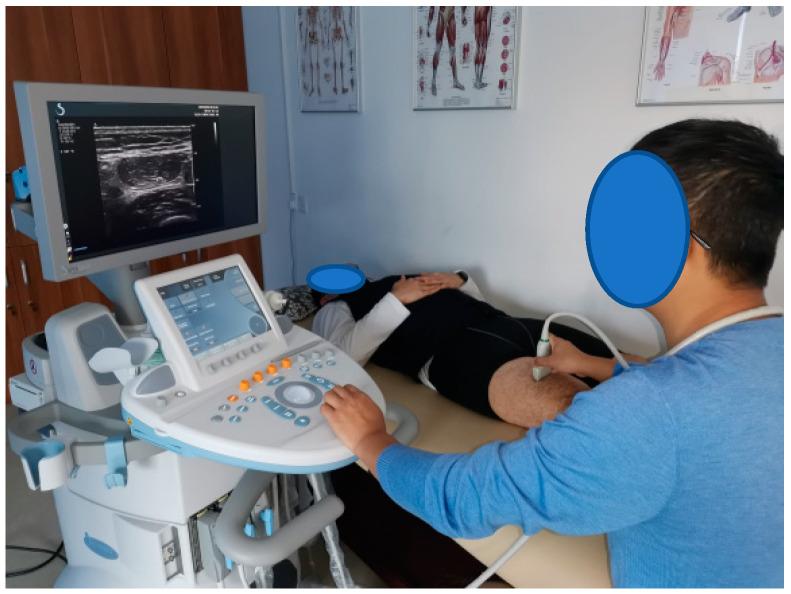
Aixplorer ultrasound imaging system.
